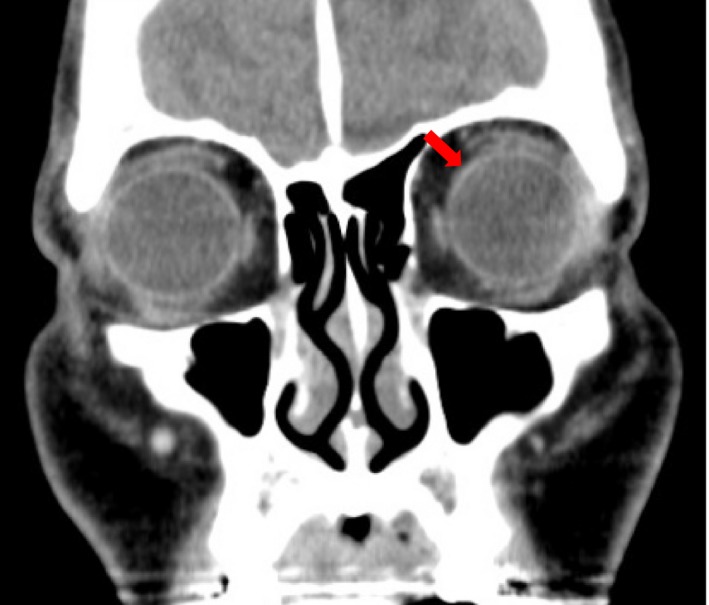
Computed tomography showing that thickness at the inner upper side of the posterior pole of the left eye becoming ambiguous after chemoradiation therapy.
